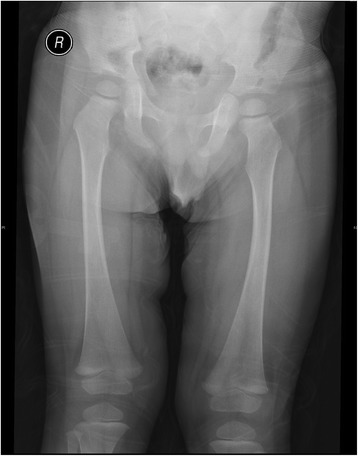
X-ray examination of patient 1 did not show skeletal involvement
